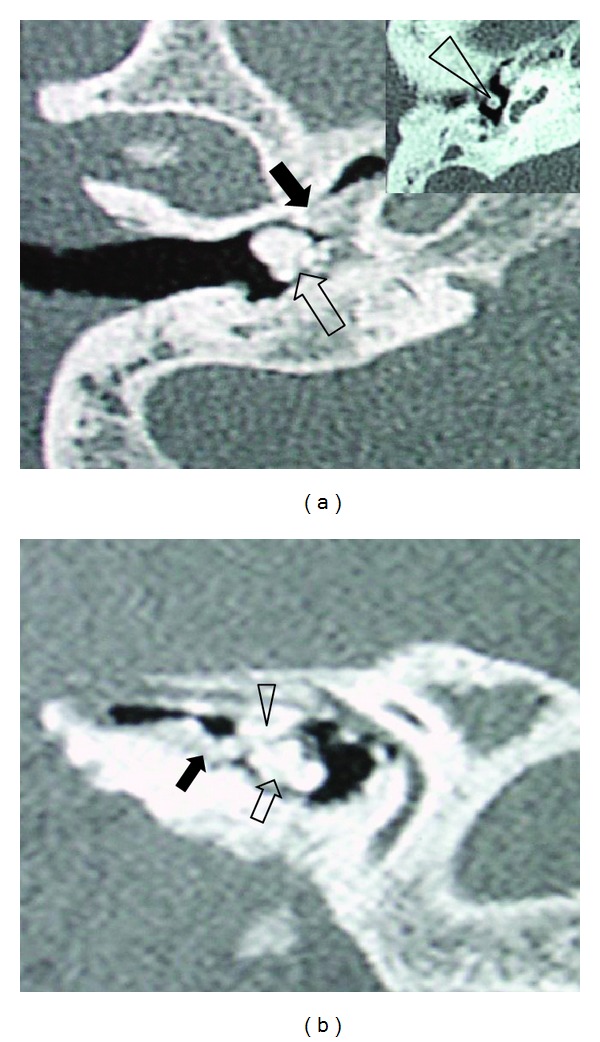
Axial (a) and parasagittal (b) computed tomography (CT) scan images of the patient. (a) One density pedunculated bony mass was on the promontory (hollow arrow), and another broad basal bony mass was on the Eustachian tube opening (black arrow), the third density bony mass located at the long crus of the incus (arrow head) (insert). (b) The density bony masses occupied the Eustachian tube opening (black arrow) and mesotympanum (hollow arrow) covering semicanal of tensor tympani muscle (arrow head).
